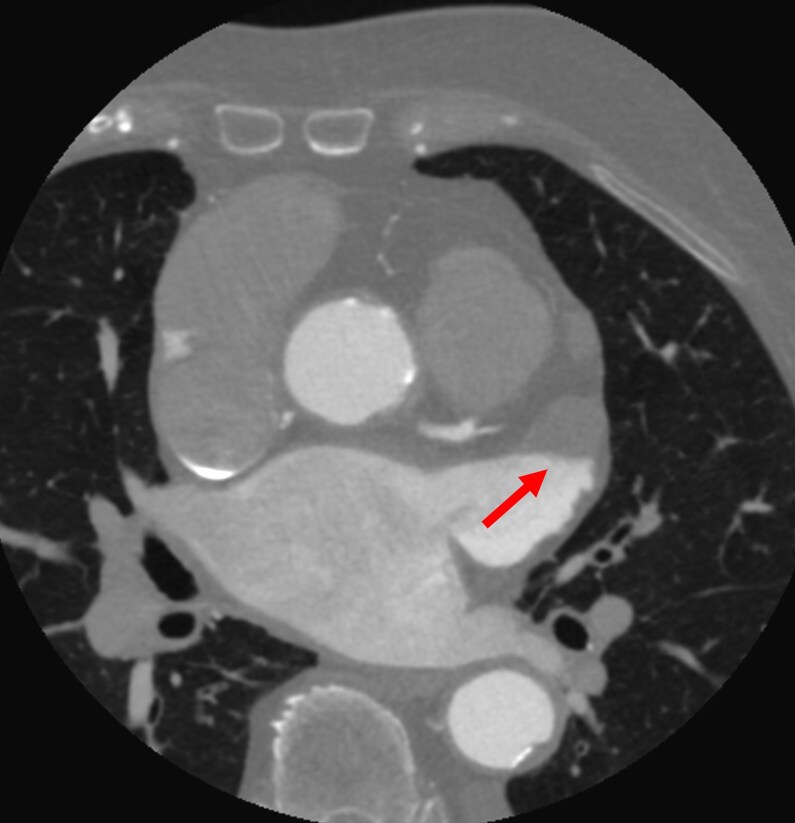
Thrombus at the bottom of left atrial appendage identified on computed tomography
